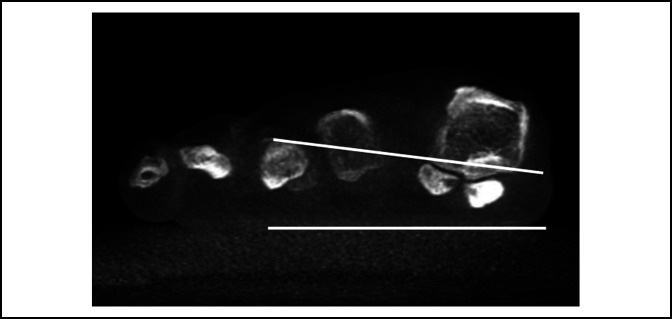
Photograph demonstrating the weight-bearing CT scan of the same patient. There is no subluxation of the lateral sesamoid. Two lines have been drawn to delineate the floor and the metatarsosesamoid facets, which demonstrate the pronation of the metatarsal bone. Owing to the pronation, when looking on the anterioposterior radiographic image, a seudosubluxation of the sesamoids is seen, and we start to perceive a roundness of the lateral aspect of the metatarsal head.
